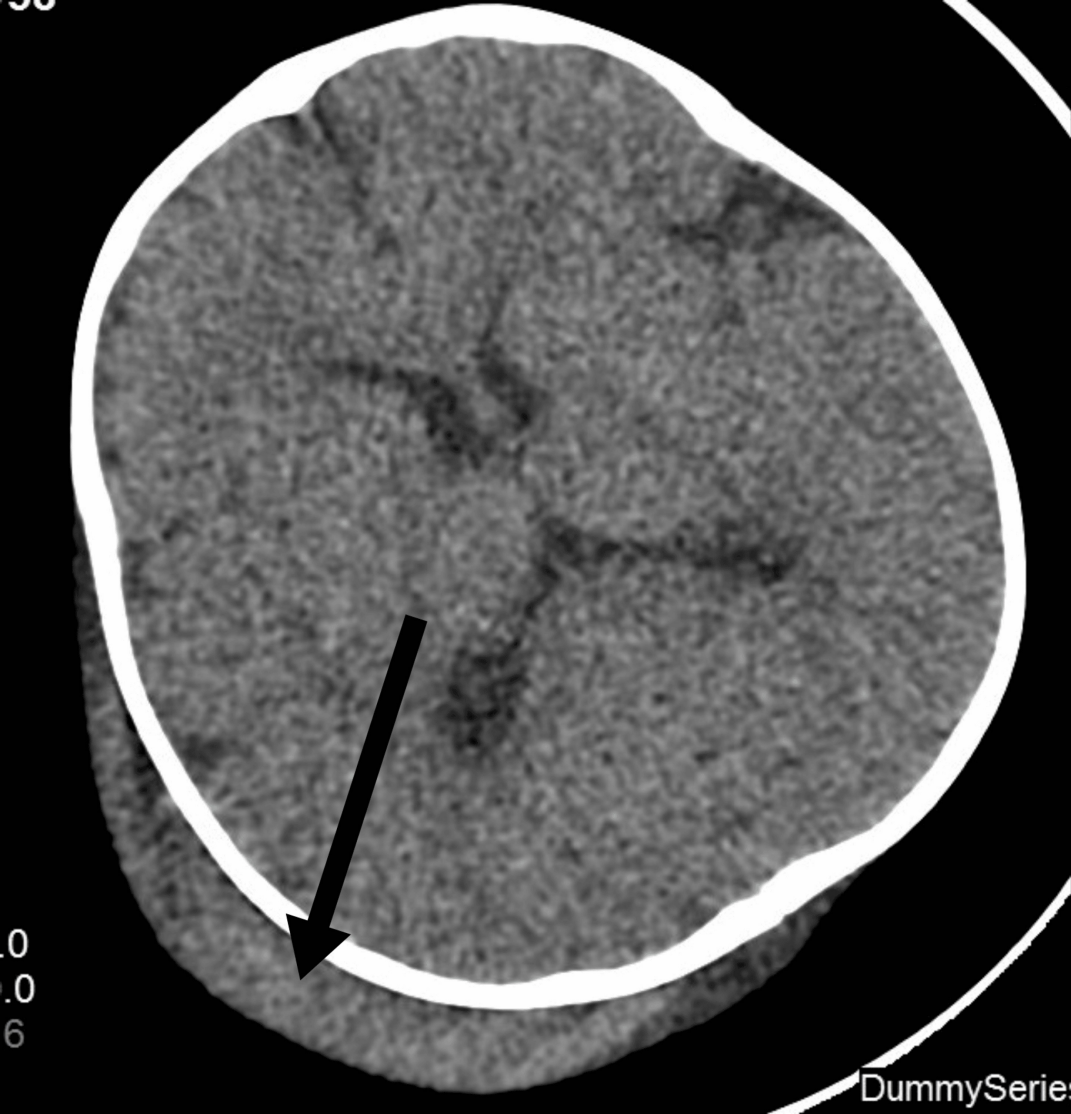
Axial CT scan of the brain revealed right parieto-occipital subgaleal hematoma (black arrow)
